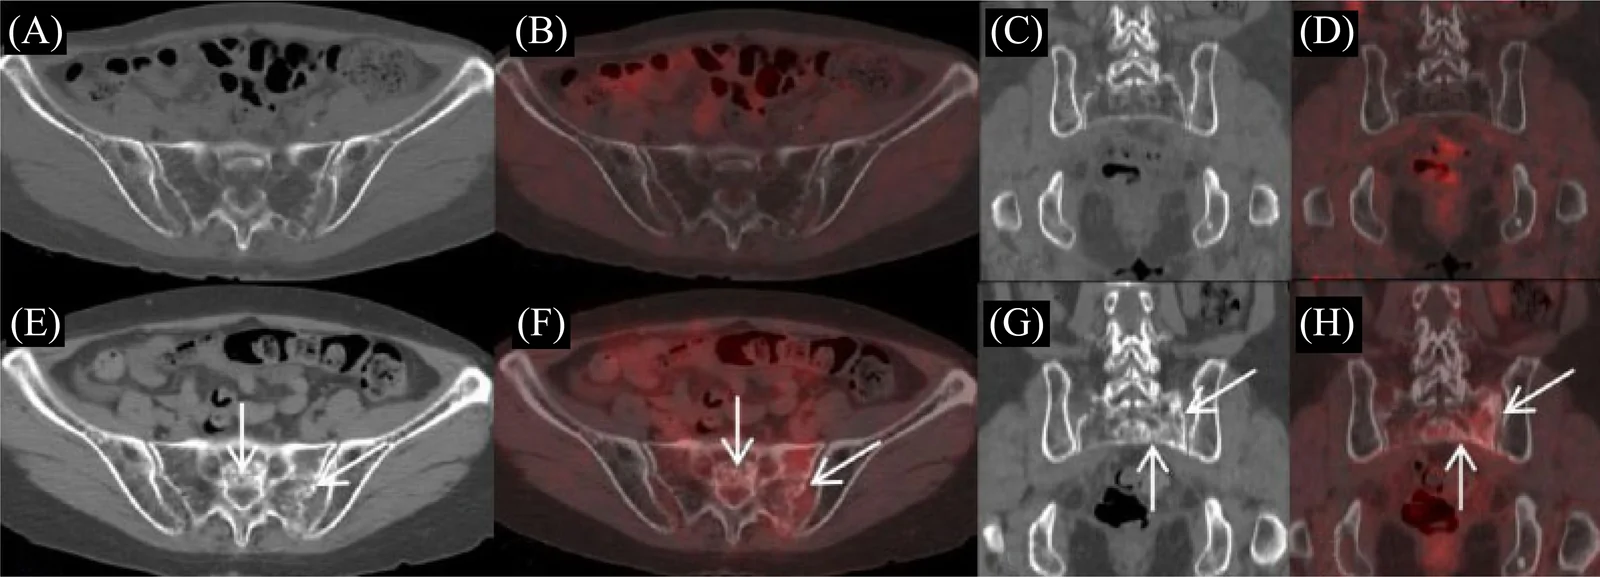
A 68-year-old female with SIFs at 30 months after radiotherapy for cervical cancer, presenting with hip pain for more than 1 month. At 10 months post-radiotherapy, axial and coronal CT images (A, C) showed decreased bone mineral density of the pelvis, presenting as osteoporotic changes, and the corresponding axial and coronal PET/CT fusion images (B, D) revealed no abnormal increased FDG uptake. Axial and coronal CT images (E, G) at 30 months post-radiotherapy demonstrated irregular low-density fracture lines in the left sacral ala and sacral body, accompanied by multiple ill-defined patchy slightly hyperdense/high-density shadows in the surrounding area (arrow, ↑), while the corresponding axial and coronal PET/CT fusion images (F, H) showed diffusely mild increased FDG uptake with a SUVmax of 3.0 (arrow, ↑).
